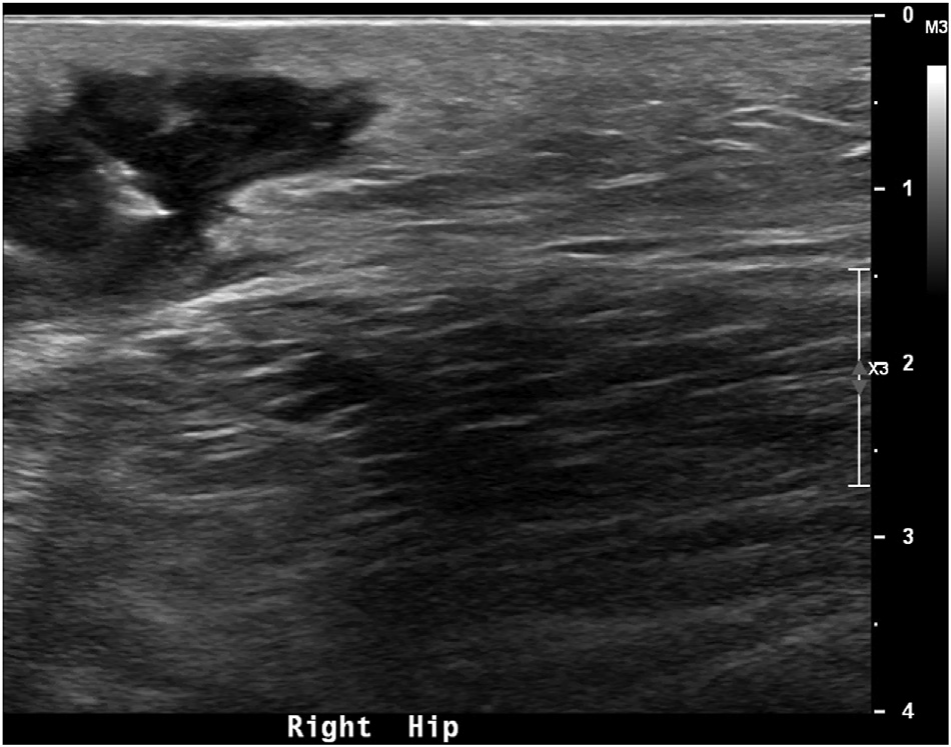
Ultrasound imaging of the right hip showing a complex fluid collection suggestive of haematoma or abscess.
